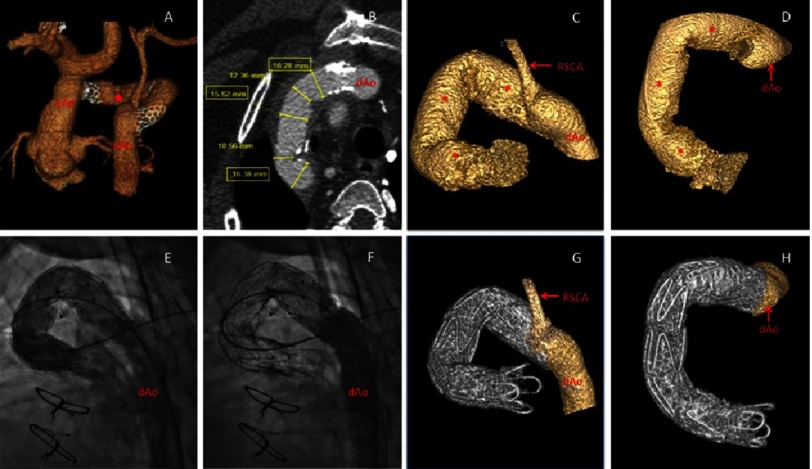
A 19-year old with complex adult congenital heart disease including interrupted aortic arch type B2 post repair with interposition graft now with dilated segments concerning for loss of structural integrity, stenosis, and folding. A, CT angiogram 3D reconstruction shows repaired aortic arch with interposition graft (⧫). Previously placed proximal and distal stents are visible with stenosis. B, CT axial image of the interposition graft showing stenosis and ominous dilated segments. C–D, Pre-intervention 3D rotational angiography (3DRA) was used to delineate complex anatomy, determine optimal imaging angles, and plan endovascular approach. Areas of dilation (*). E, Fluoroscopic contrast angiogram post endovascular graft (Cook Medical, Bloomington, IN) deployment in optimal working angle derived from 3DRA. F, Repeat angiogram post bare metal stenting within the endovascular graft. G–H, Post-intervention 3DRA shows endovascular repair of previously compromised interposition graft.
